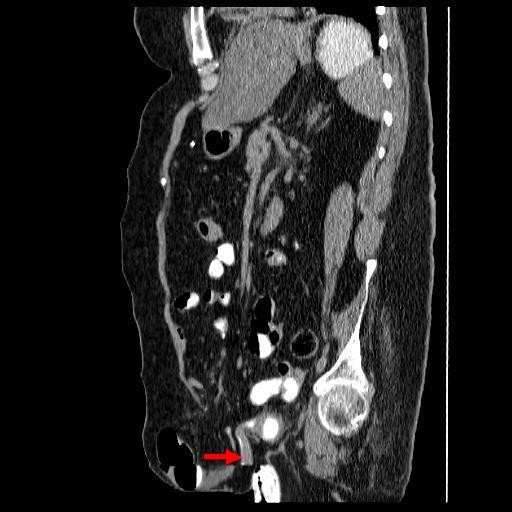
Oblique sagittal reformat of computed tomography of abdomen, performed on 19 June 2007 revealed herniation of vesicostomy. (arrow).
